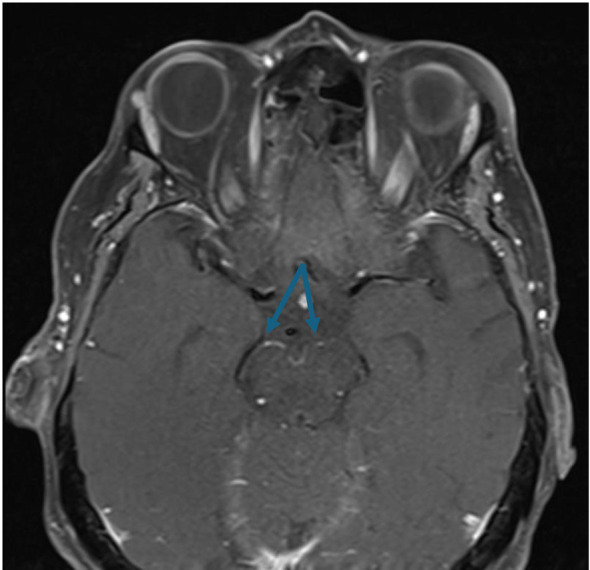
T1 MRI brain and orbits with contrast demonstrated mild enhancement at the proximal middle cerebral arteries (MCA) bilaterally, as indicated by the blue arrows. This finding was suggestive of a vasculitic process prior to the patient’s presentation to our clinic.
